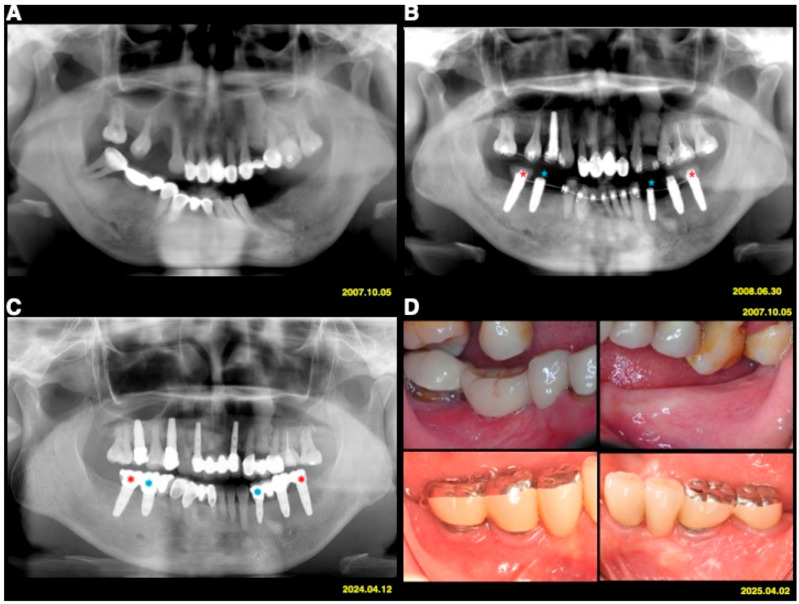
Case 2 (A) A 40-year-old female patient with Stage IV periodontitis. (B) Implants served as orthodontic anchorage (test; red stars) and for prosthetic rehabilitation only (controls; blue stars). (C) Radiographs in 2024 showed minimal periodontal and peri-implant bone loss. (D) Clinical findings at 17.5 years post-treatment demonstrated stable and healthy peri-implant tissues.
